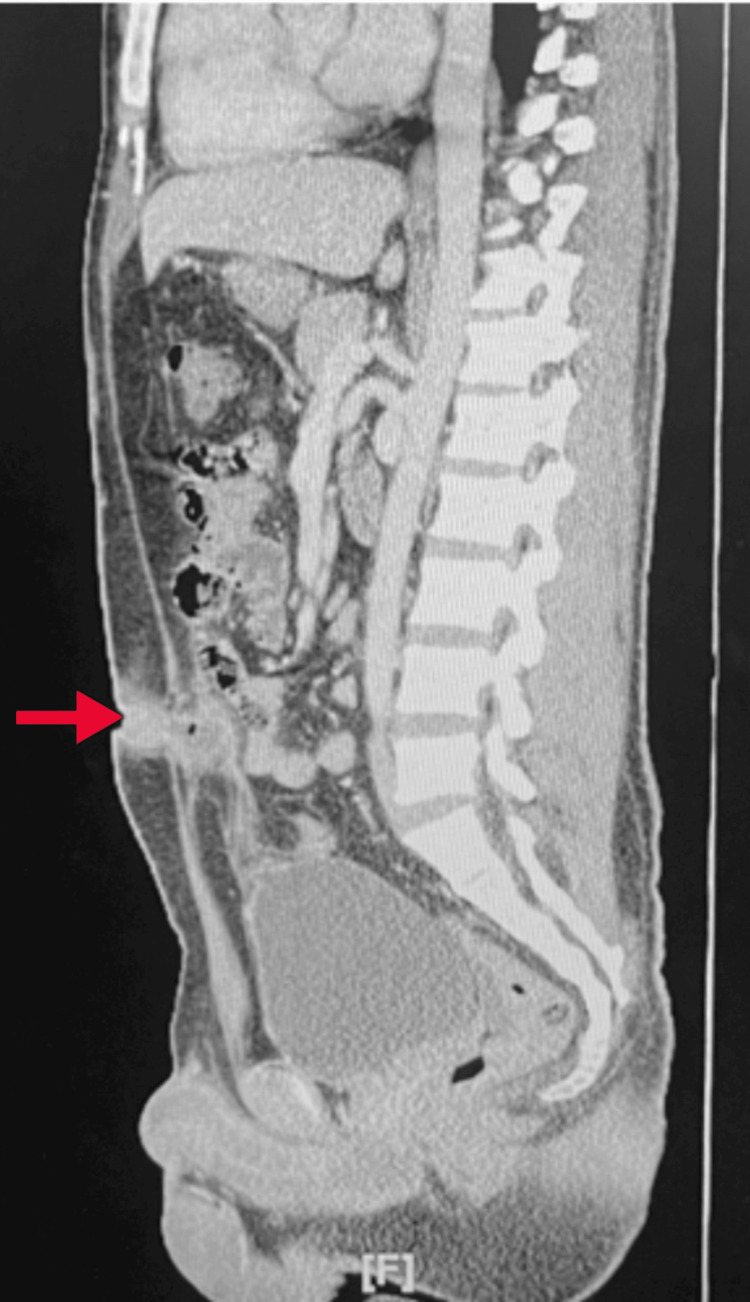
Sagittal section CT abdomen pelvis
